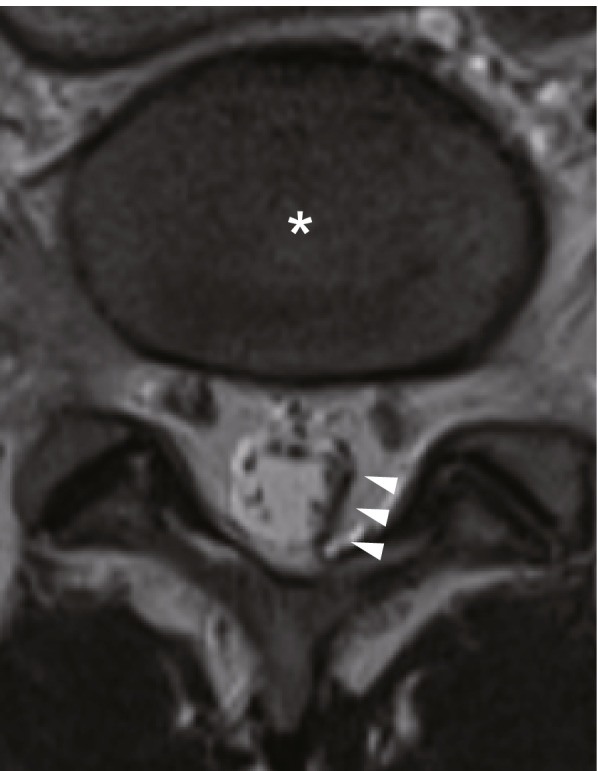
Magnetic resonance imaging of the lumbar spine 3 days after spinal anesthesia. The left L5/S level shows a T2W1 low signal (white arrowheads). Adhesions between the proximal nerve roots and the cauda equina are also noted. There is no abnormal signal in the spinal cord. The asterisk indicates an intervertebral disk at L5/S1
